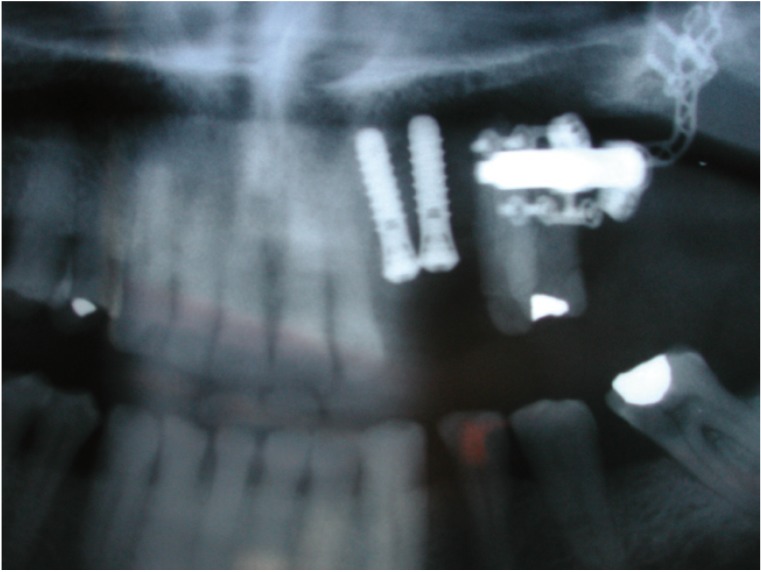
Detail from orthopantomograph image showing implants placed in newly generated bone before osteodistractor removal.
